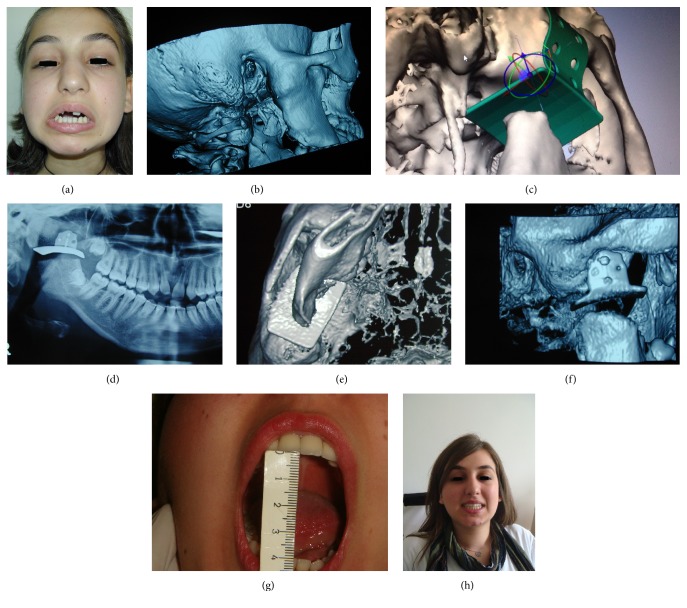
(a) The patient (Case  1) is not able to open her mouth due to reankylosis. (b) CT reveals reankylosis at the right TMJ five years after the first surgical treatment. (c, d) Panorex shows horizontal resection of the ramus via subankylotic approach. (e) The prosthesis creates a barrier to prevent reankylosis. (f) Complementation of the neoformation of the condyle two years after surgery. (g) Mouth opening, six years after surgical treatment. (h) Facial appearance after surgical and orthodontic treatment.
